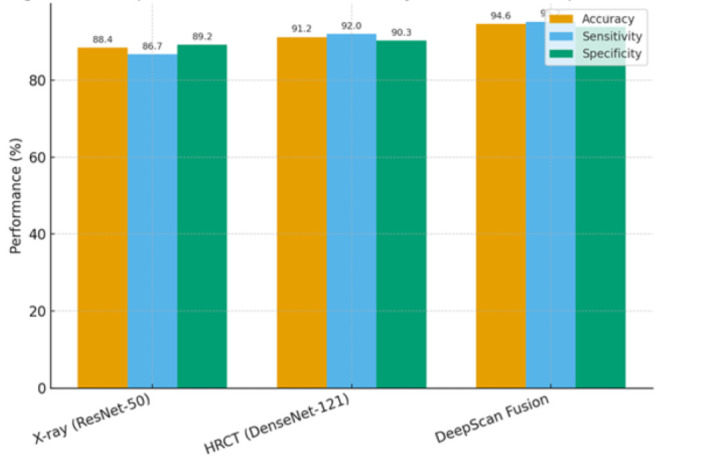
Comparative performance metrics of X-ray, HRCT and Deep Scan models.
